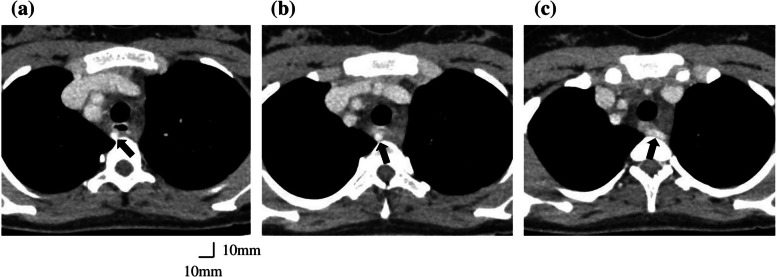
Thoracic contrast-enhanced computed tomography images. (→): Aberrant left subclavian artery
